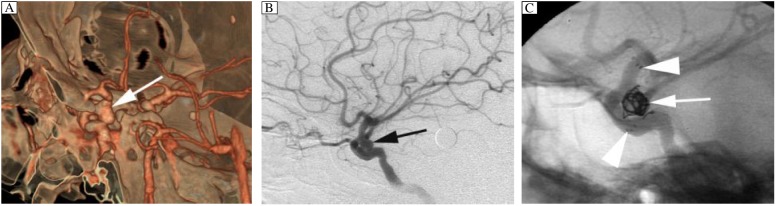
Right posterior communicating artery aneurysm in a 59-year-old woman with SAH. A: VR image from CTA clearly shows the broad-necked aneurysm (arrow), and indicates that the aneurysm should be coiled with a stent-assisted technique. B: the broad-necked aneurysm was proved by DSA images (arrow). C: The broad-necked aneurysm was treated with a stent implantation (arrowhead). SAH: subarachnoid hemorrhage; VR: volume rendering; CTA: computed tomography angiography; DSA: digital substraction angiography.
